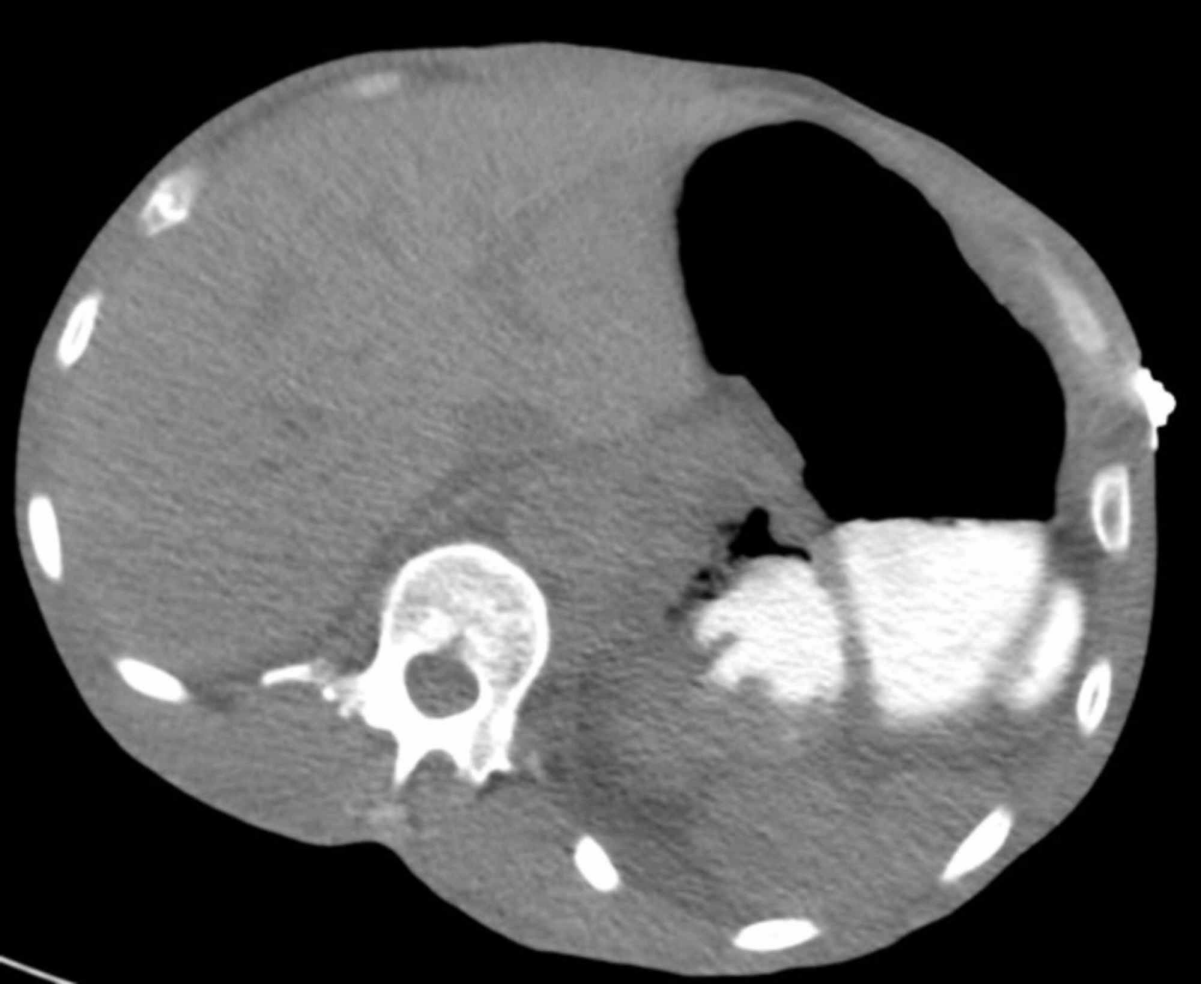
Contrast CT of the abdomen with large necrotic renal mass and presence of oral contrast in the necrotic renal mass.
